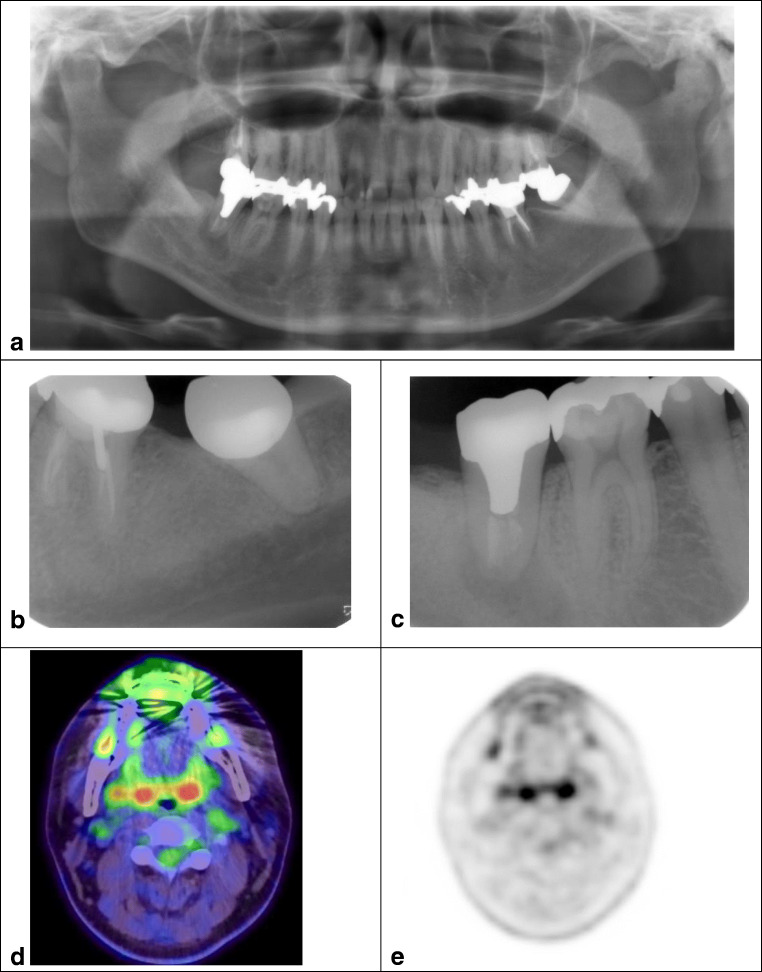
Representative cases. A 30-year-old male with external auditory canal cancer after receiving heavy ion therapy. He had negative clinical symptoms in the oral cavity when visiting our department. The lesion was found at the right mandibular second molar root apex. Panoramic radiography (a), dental radiography (b, c), and ^18^F-FDG-PET/CT with PAD score grade 3; right lesion (d, e)
